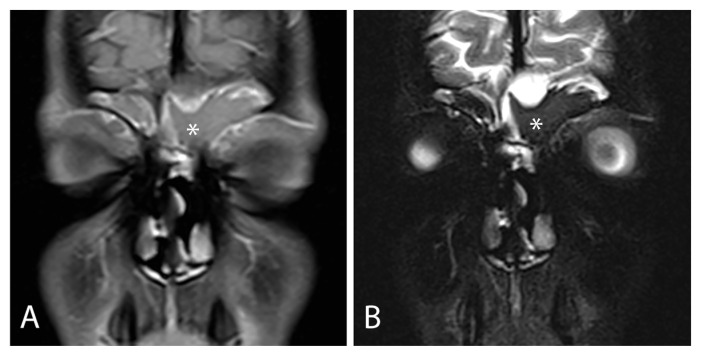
(A) Contrast-enhanced MRI images in T1-weighted and (B) T2-weighted sequences showing chronic rhinosinusitis complicated by a left fronto-ethmoidal mucocele (marked with white asterisks). Parent/guardian consent to use clinical pictures of the patients was obtained.
